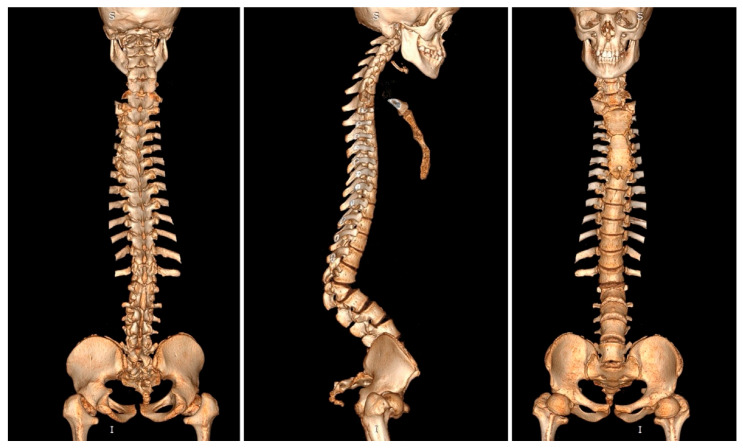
Preoperative 3D-CT of a 16-year-old girl with achondroplasia and congenital severe kyphosis.
